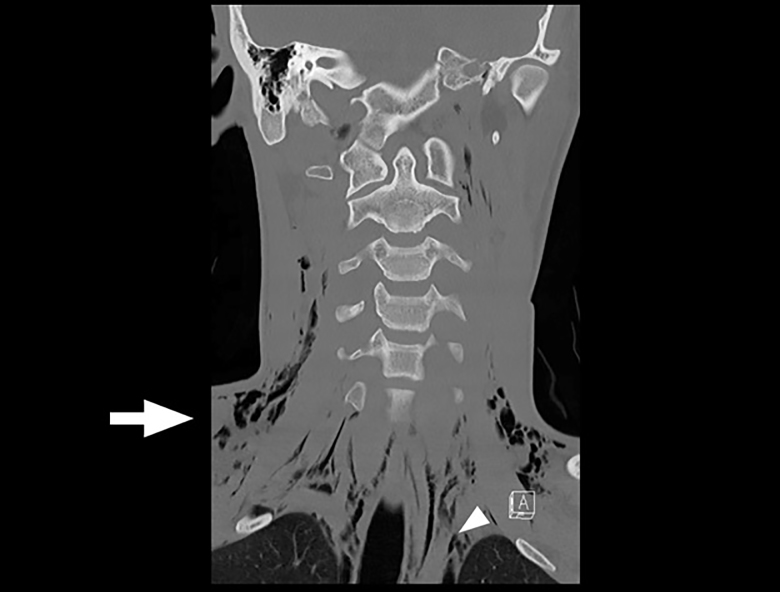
Coronal view of computed tomography of the patient’s neck demonstrates subcutaneous emphysema (arrow) and pneumomediastinum (arrowhead).
